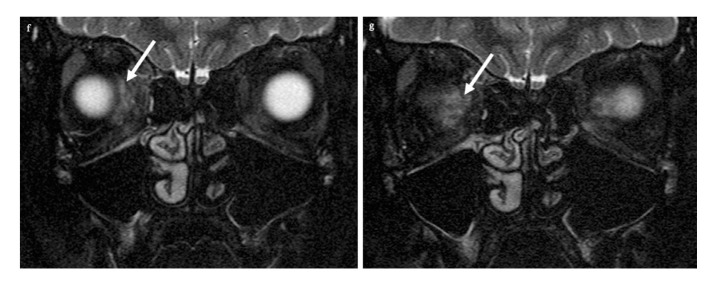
Examination of the right eye revealed a superficial conjunctival wound nasally and a dilated pupil (a). On the MRI scan, there is loss of the subarachnoid space in the right optic nerve (arrows in panel (b): the subarachnoid space of the left optic nerve is normal). The respective transverse T2-weighted image shows a central signal increase in the optic nerve (arrow in (c)). On the diffusion-weighted imaging, a punctuate signal increase in the optic nerve in the same location is seen (arrow in (d)); the corresponding ADC map shows signal loss in the same location (arrow in (e)). The globe as well as the left eye and orbit are unchanged, while there is a diffuse signal increase (arrows) in the right retrobulbar space nasally (f,g).
